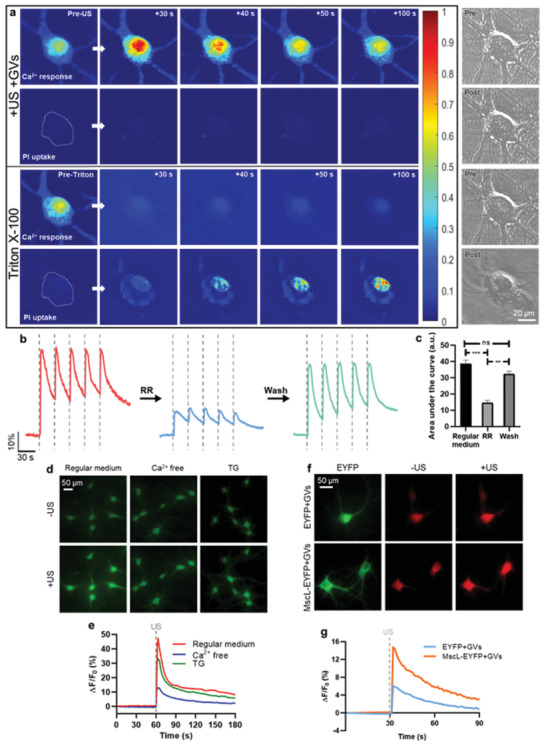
Mechanosensitive ion channels are an important mechanism for GV‐mediated ultrasound stimulation. a) Upper: Calcium response and PI uptake (indicating membrane integrity) during US+GVs stimulation. Lower: Calcium response and PI uptake (indicating membrane integrity) following addition of 0.2 mm Triton X‐100 as a positive control for loss of membrane integrity. Right: Brightfield images of the images cells before and after ultrasound + GVs or Triton X‐100. All images shown in this panel are representative. b) Time‐resolved calcium responses of neurons during US+GVs stimulation, first as normal, then in the presence of mechanosensitive ion channel blocker ruthenium red (RR), and then after RR was washed away. c) Quantification of area under the curve before, during and after RR treatment as shown in (b). Bars represent the mean ± SEM of three independent experiments. *p* < 0.01, p* < 0.001, one‐way ANOVA with post‐hoc Tukey test. d) Representative images of calcium responses of neurons in regular medium, Ca^2+^‐free solution and Thapsigargin (TG). e) Time‐course calcium imaging of cells before and after ultrasound + GVs stimulation in the three solutions indicated in (d). f) Primary cultured cortical neurons expressing MscL‐G22S‐EYFP or EYFP were exposed to a 100 *μ*s pulse of 0.13 MPa ultrasound. Representative images of X‐Rhodamine fluorescence before and after ultrasound, as well as EYFP expression are shown. g) Time‐course imaging of the results shown in (f).
